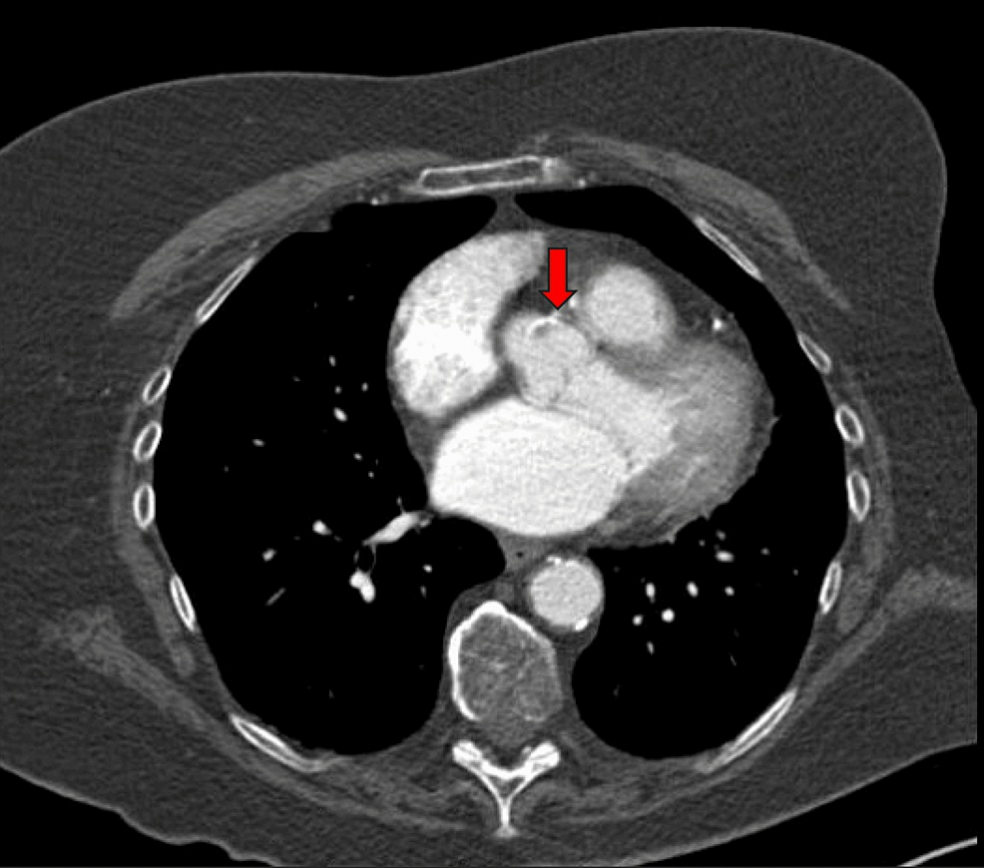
Computed tomography (CT) of the chest with contrast in axial view showing mixed-density atherosclerotic plaque at the origin of the right coronary ostium
